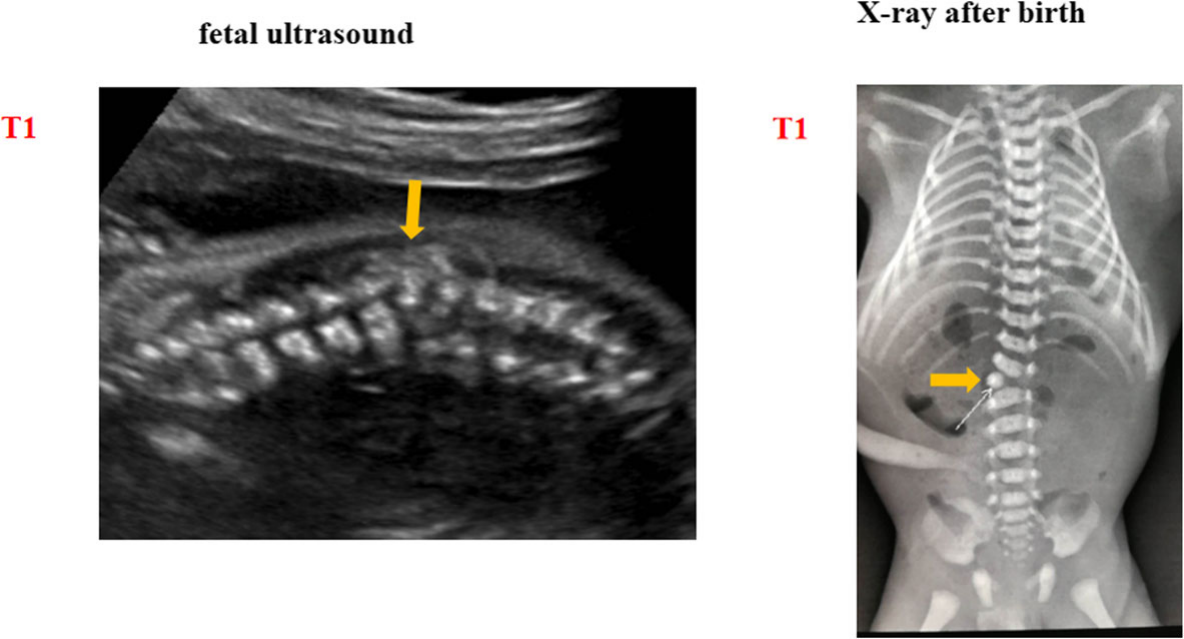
Imaging manifestations of the DCDA twins in case one. Panel 1 shows the fetal ultrasound of the congenital hemivertebrae twin (T1), and panel 2 shows the X-ray after the birth of T1. The yellow arrow indicates the defect of fetal hemivertebrae. T1 is short for the congenital hemivertebrae twin in case one
